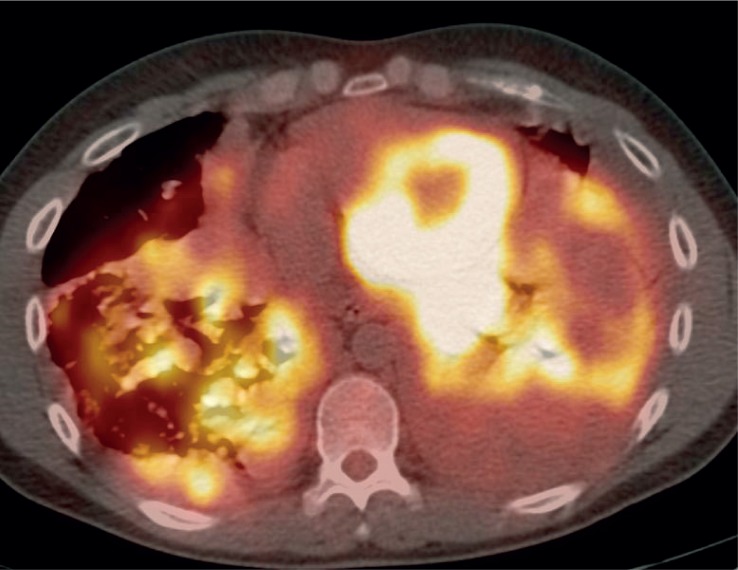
Axial FDG-PET/CT also showing significant FDG uptake in pulmonary and pleural metastases.
